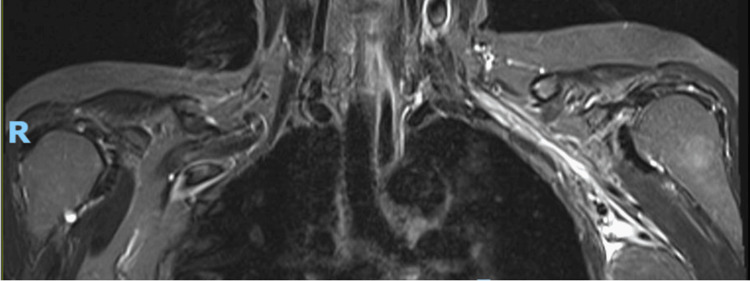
MRI brachial plexus: coronal fat-suppressed T2-weighted image showed abnormal thickening, T2 hyperintensity, and enhancement involving the left-sided trunks, divisions, and cords of the brachial plexus
